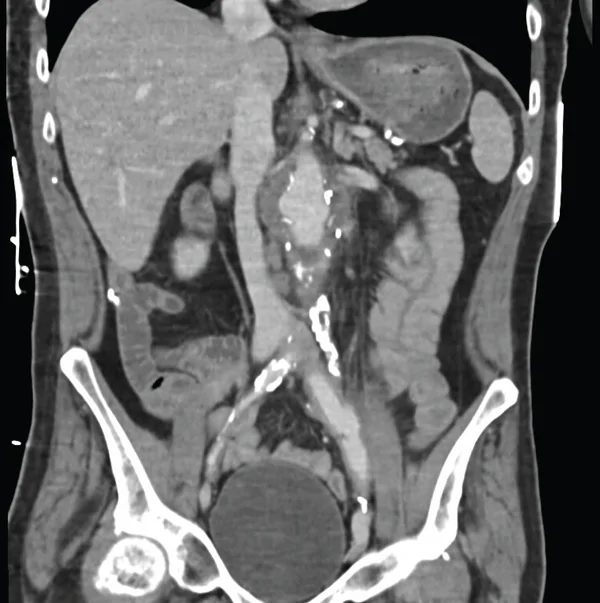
CTA of the abdomen and pelvis that shows slight increase in periaortic induration without evidence of contrast pooling or active bleeding.
